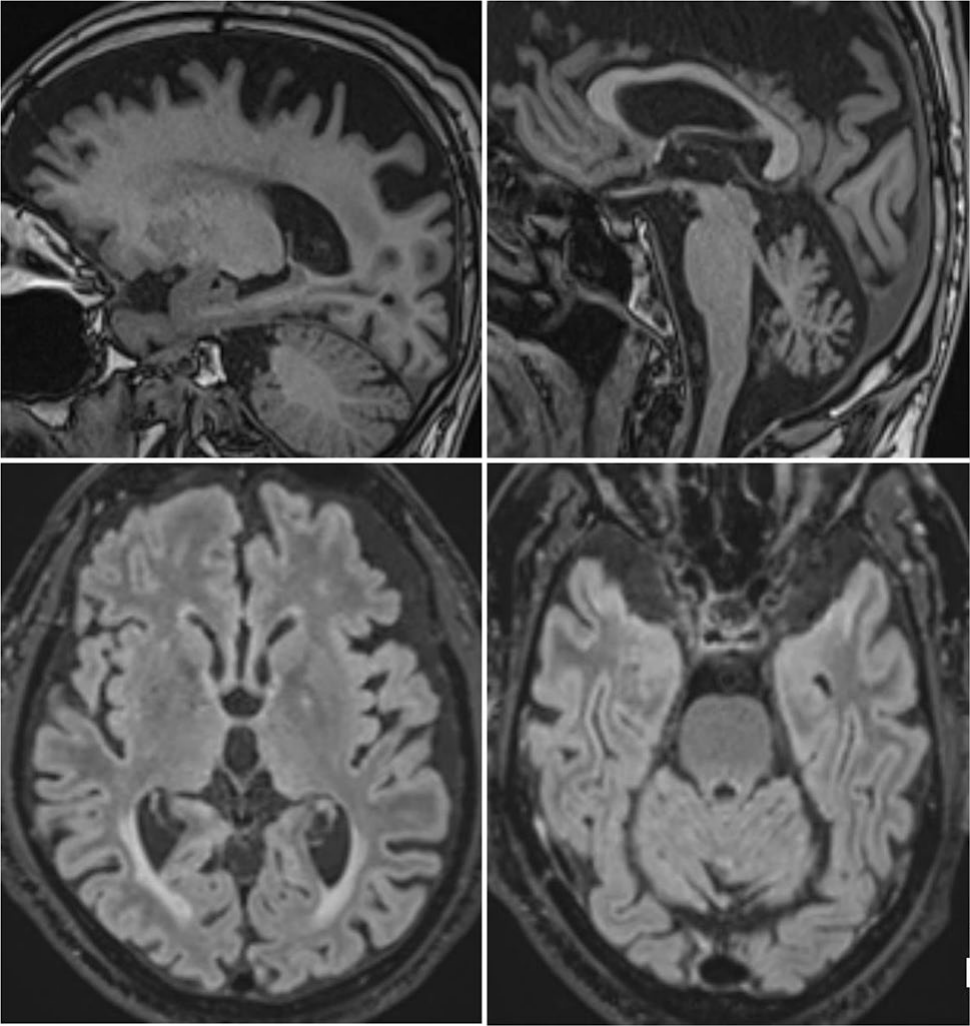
New brain MRI of the patient. Sagittal MRI-images (MPRAGE) and axial FLAIR-weighted images of our patient showing frontoparietal cortical atrophy and cerebellar, vermis-accentuated atrophy
